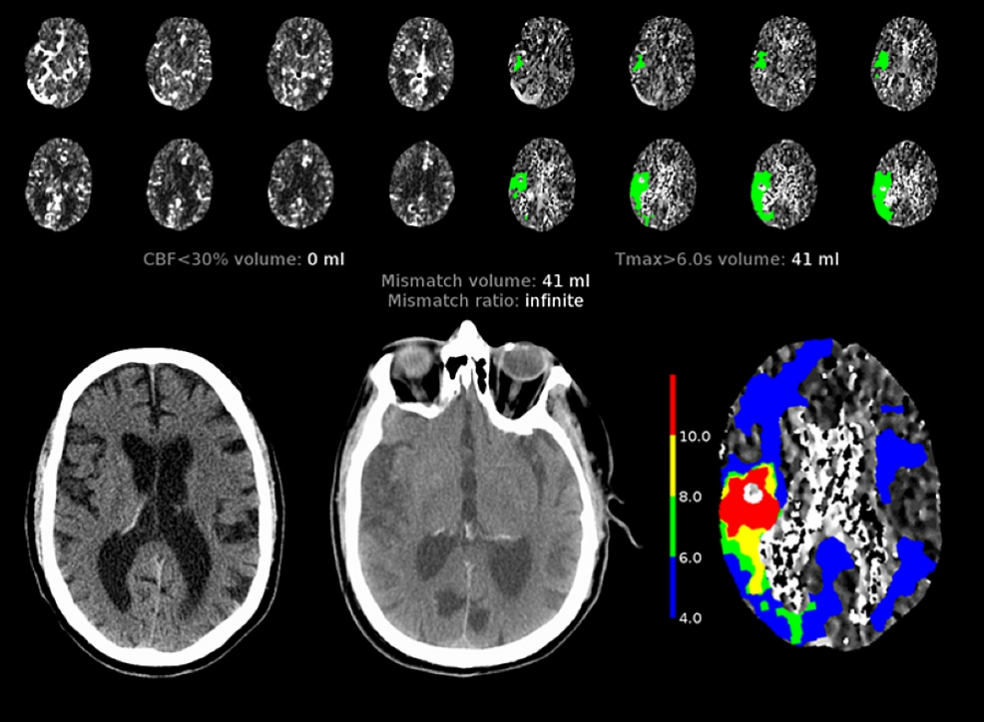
Scans of Patient A Patient A’s initial CT perfusion scan (top row) showed a moderate-sized 41 ml penumbra in a right MCA branch territory. The infarcted area is not visualized on the initial CT head (bottom row, left) while the final infarct in the right temporal region can be seen in the final CT scan (bottom row, middle). The final infarct volume corresponds to the Tmax > 10.0 s (bottom row, right) of 15 ml.
